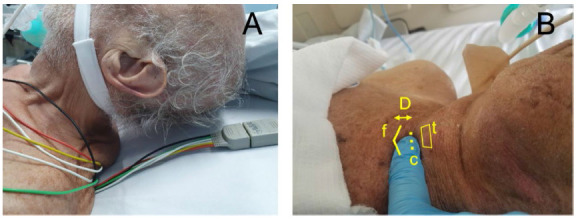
Some factors that may preclude ultrasound-guided percutaneous tracheostomy: Limited neck extension (A); and in B, and short distance (D) between the crichoid (c) and the sternal notch (f); Thyroid cartilage (t).
